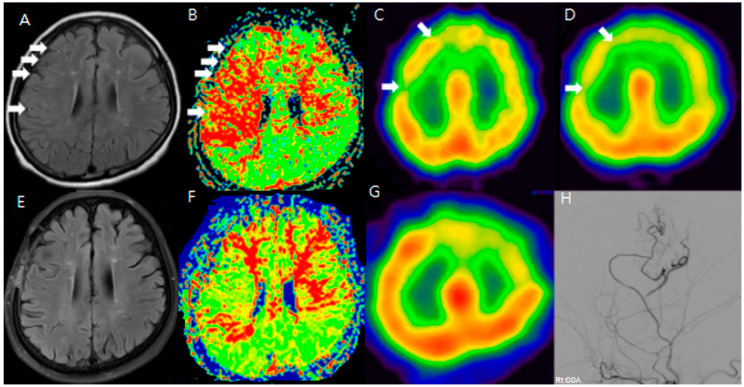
F/64 Moyamoya disease patients. Preoperative (A–D) and postoperative (E–H) MR FLAIR, perfusion MR, and SPECT show improved HV and CVR after STA-MCA bypass (white arrow: hyperintense vessels and decreased CVR in the same region). The areas showing hyperintense vessels on FLAIR (white arrows in (A)) correspond to zones of diminished CVR on SPECT (B–D). After STA-MCA bypass, the reduction in HV (E) is associated with improved perfusion, suggesting that the ivy sign reflects dynamic cerebrovascular status (F–H).
